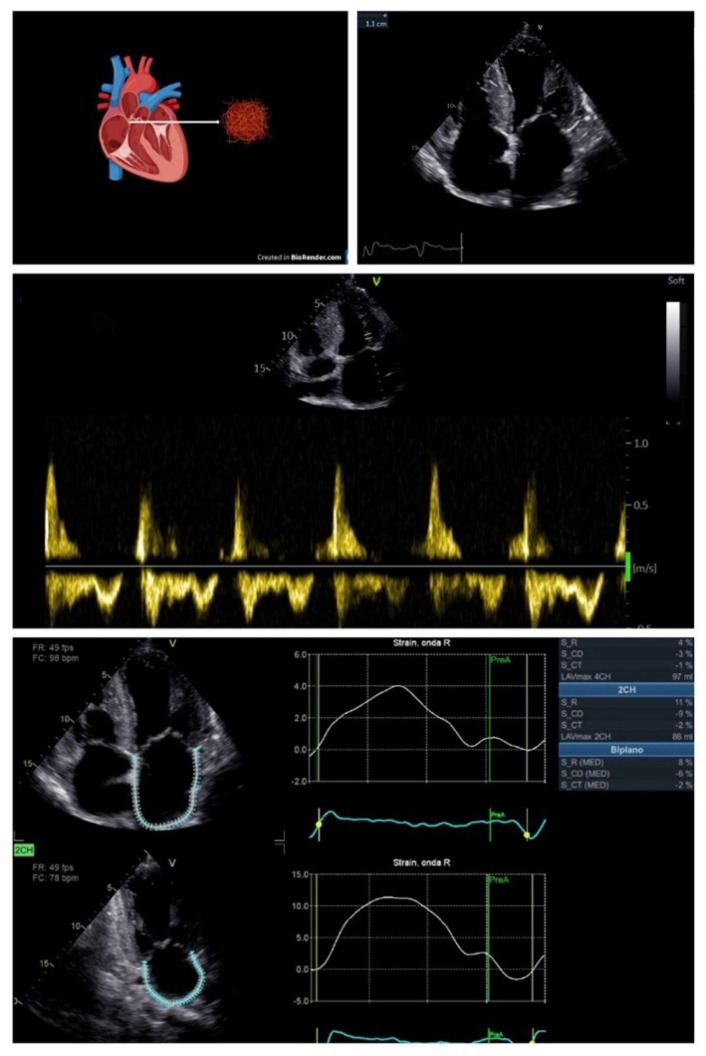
Atria infiltration by misfolding proteins causes the increase of interatrial septum thickness with restrictive doppler pattern and reduced peak atrial longitudinal strain (PALS) and peak atrial longitudinal strain (PACS).
